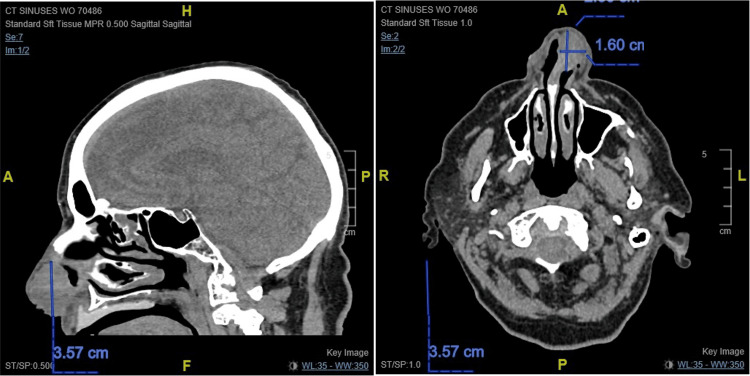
Sagittal (left) and axial (right) CT showing a 2.6 × 1.6 × 3.6 cm mass occupying the left anterior nasal cavity and eroding through the cartilaginous septum to the contralateral nasal cavity.
